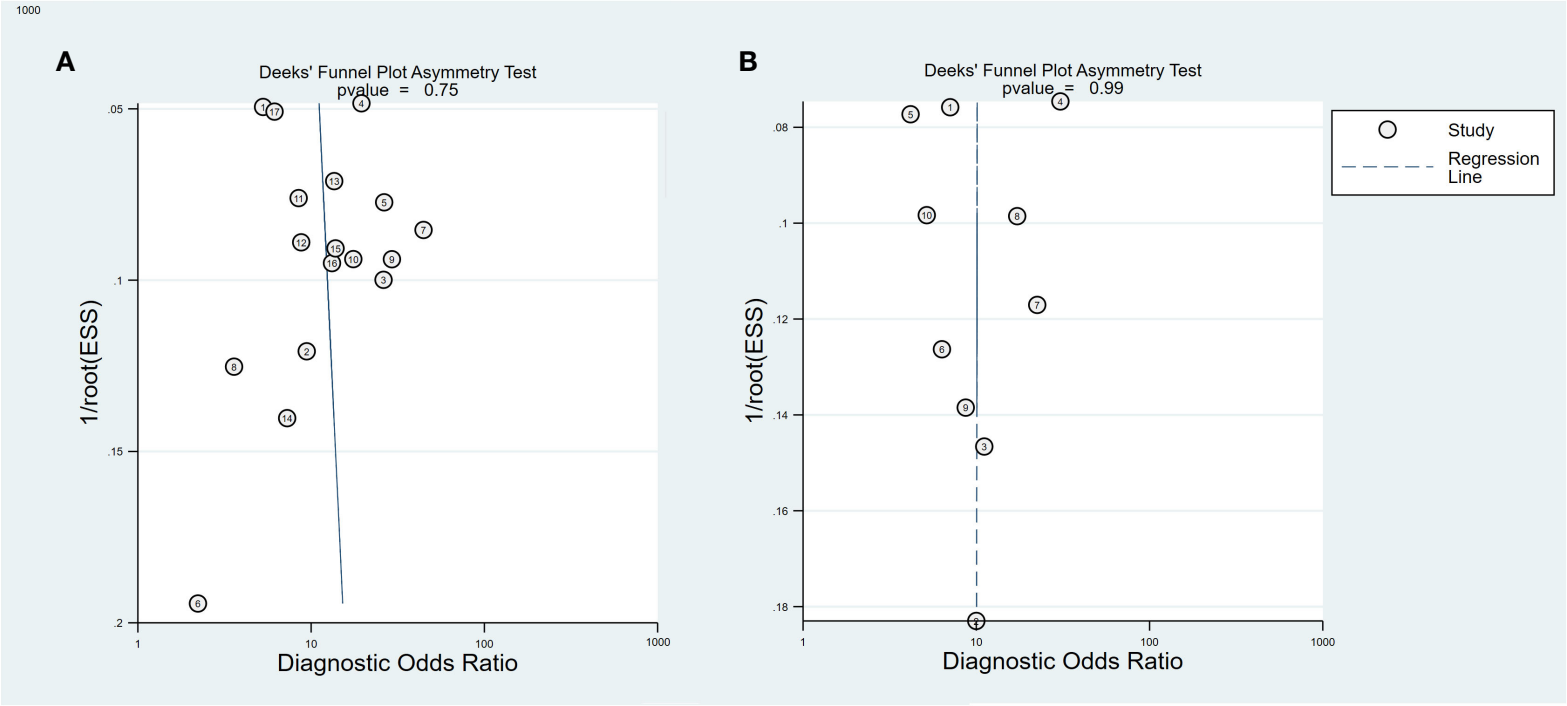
Deeks funnel plot for the publication bias test of ^18^F-FDG PET/CT radiomics for the prediction of EGFR mutation status in NSCLC, In both training (A) and validation cohorts (B).
